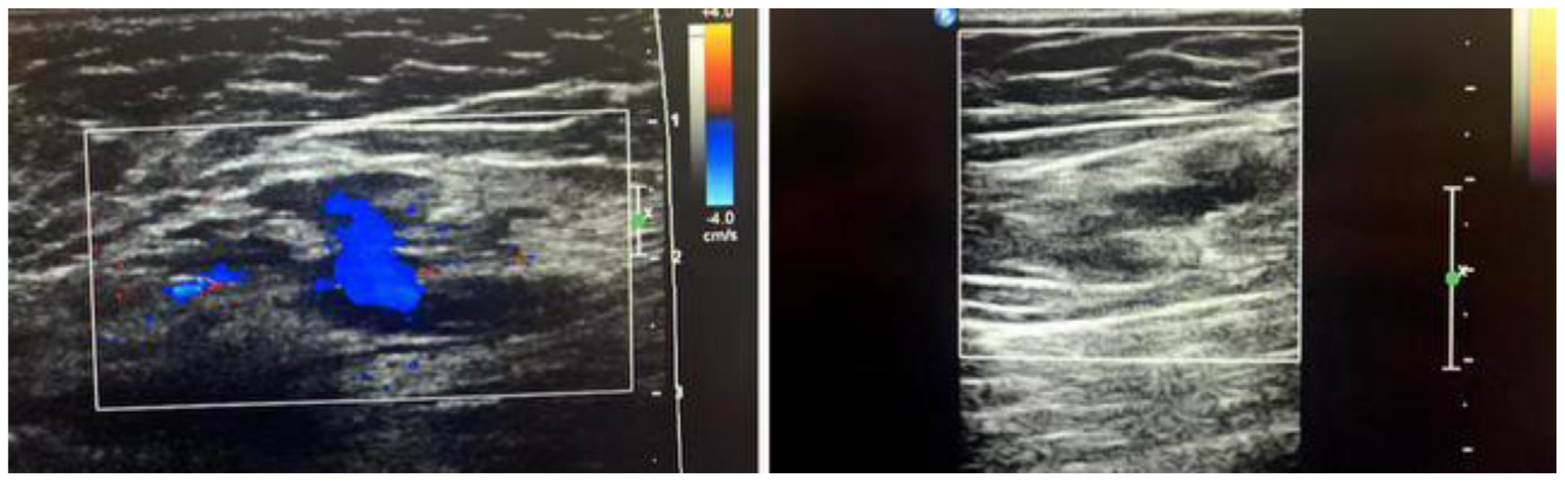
Change in intralesional blood flow seen on duplex ultrasound examination after direct stick embolization (DSE) with Yale OCR-7737. Duplex ultrasound examination of a lower extremity venous malformation before and after DSE with Yale-OCR7737. Color flow Doppler imaging performed 6 weeks after treatment demonstrates complete cessation of intralesional blood flow.
